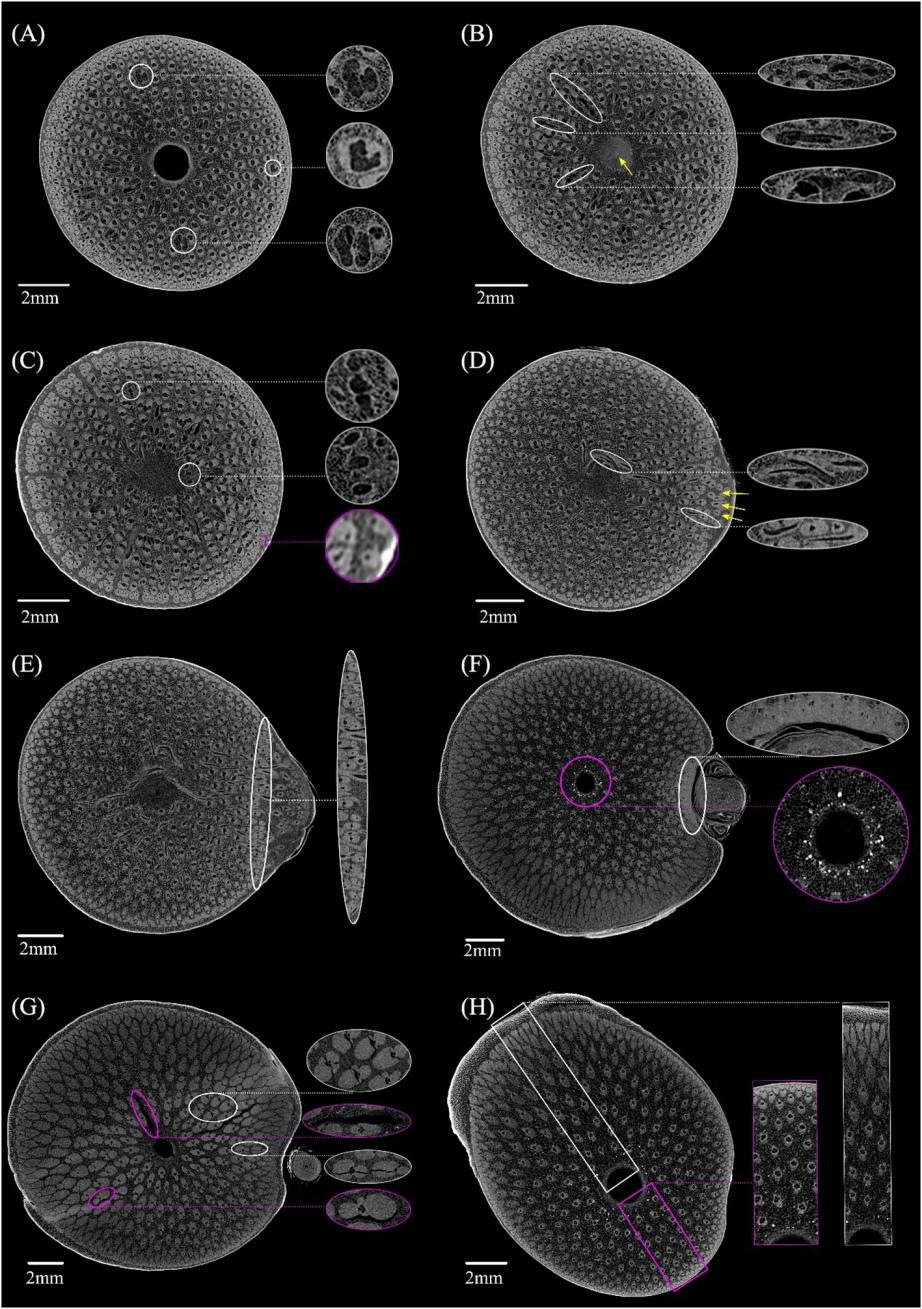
X-ray microtomography slices at different heights in the *Chimonobambusa tumidissinoda* node: (A) Slice 0: the VB with larger conducting tissue (white circle). (B) Slice 50: the TVB (white circle) and diaphragm (yellow arrow). (C) Slice110: the bifurcated small VB (white circle) and the small VB in the cortex (magenta circle). (D) Slice 300: branch (yellow arrow) and a TVB (white circle). (E) Slice 385: the boundary between the culm and the branch (white circle). (F) Slice 600: developed fibers near the branch of the culm and the cortex were not very pronounced (white circle), the starch granules (magenta circle). (G) Slice 872: shorter cracks (white circle) and longer cracks (magenta circle). (H) Slice 1292: inconsistent radial variation pattern of the VB (white box, magenta box).
